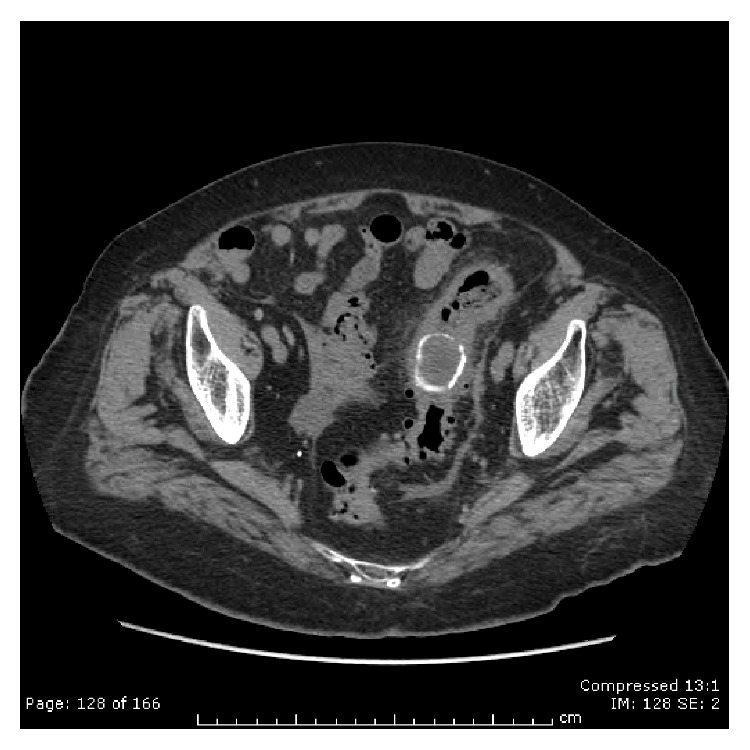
Computed tomography. Gallstone impacted in the sigmoid colon.
